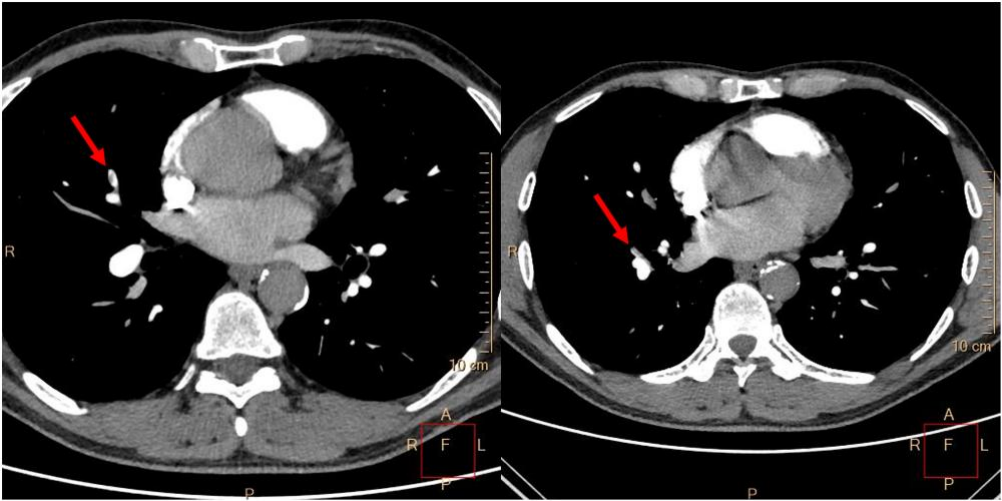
Computed tomography pulmonary angiogram: small thrombi in the fifth and eighth pulmonary segments of the right lung (red arrows).
